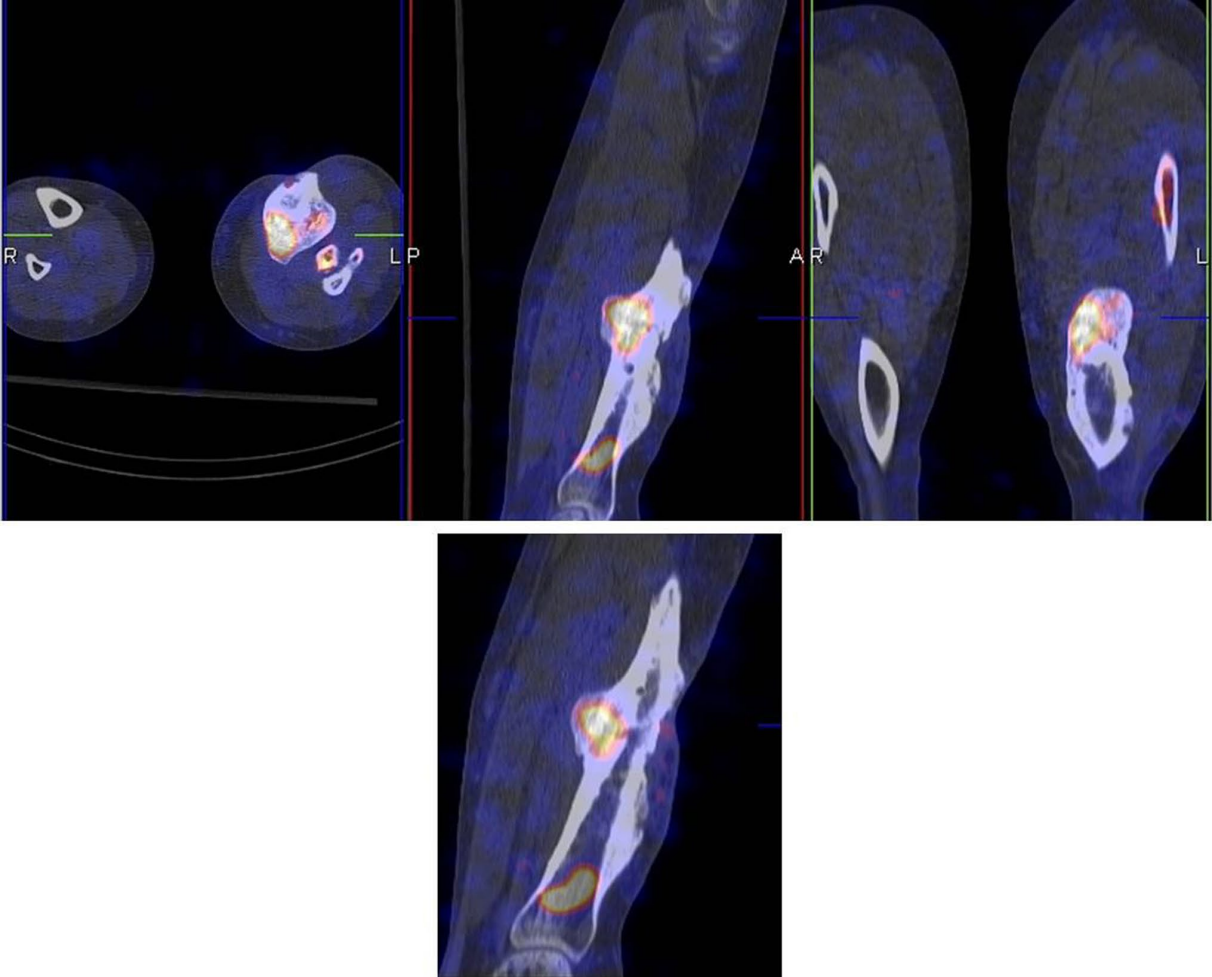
WBC SPECT-CT of patient C
